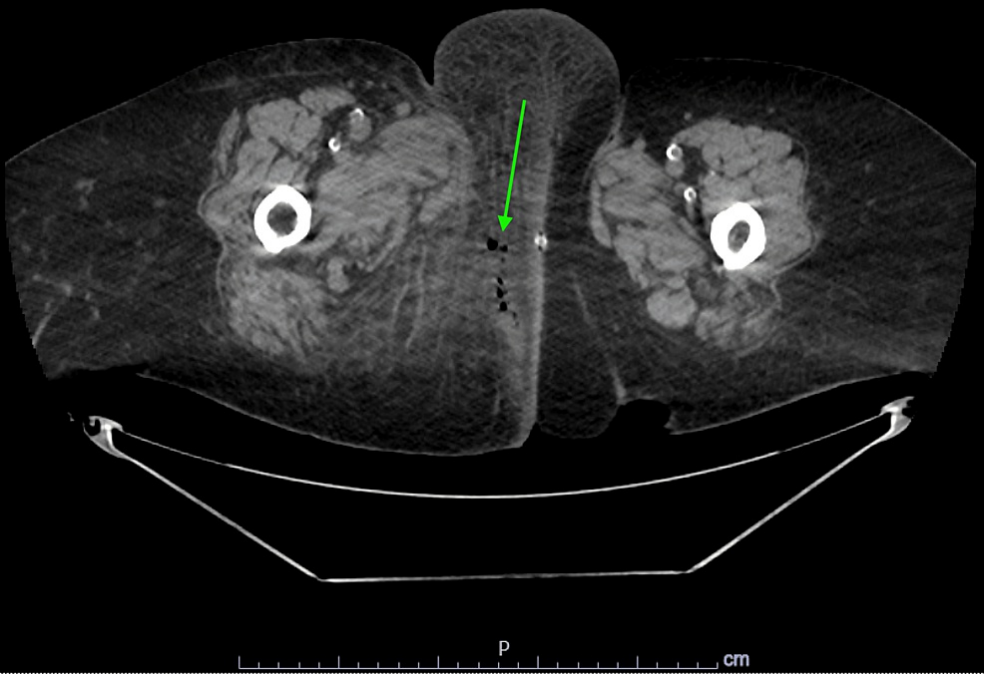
CT scan (axial cut) showing right-sided gas in the connective tissue.
